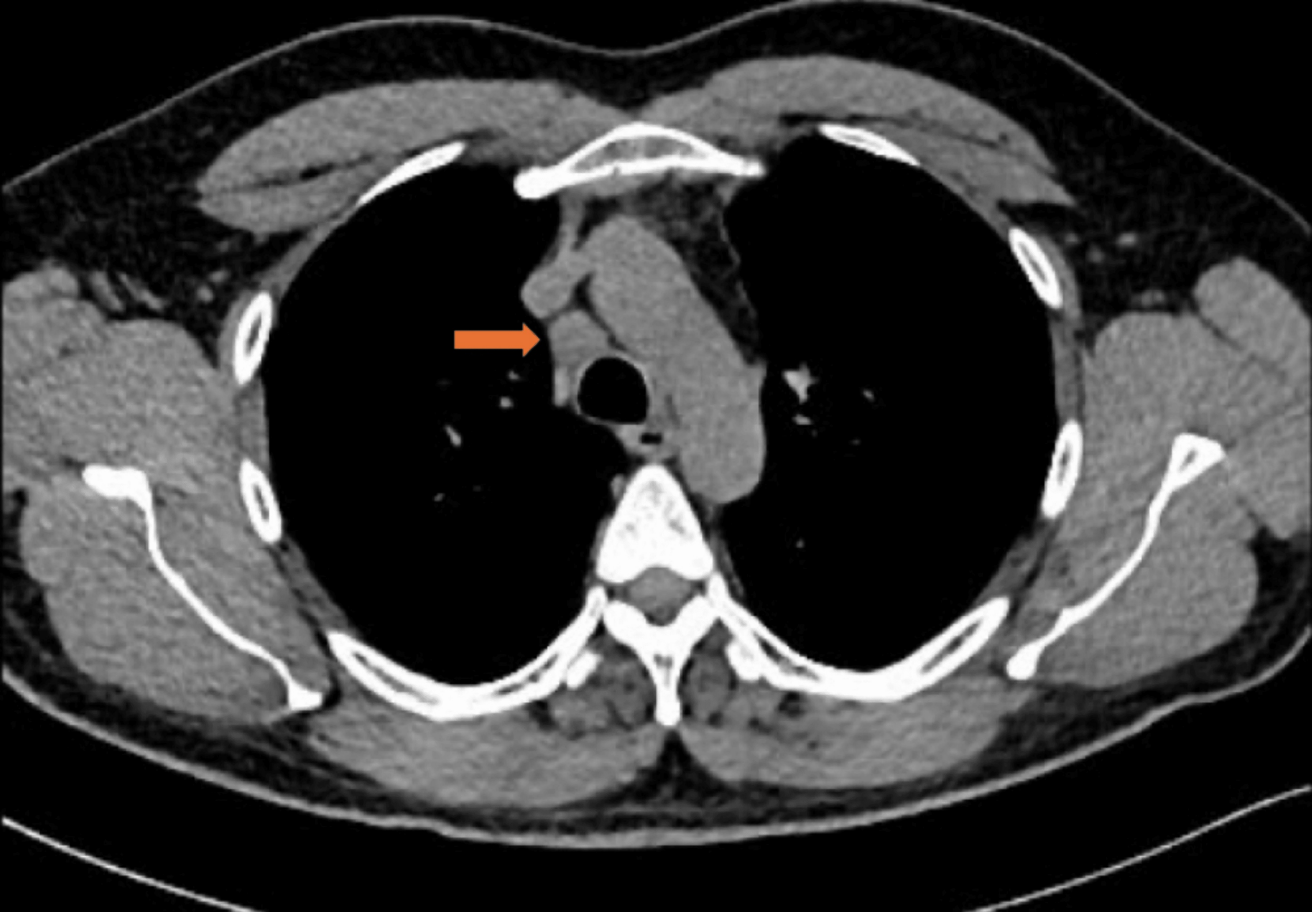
The axial image of the chest CT in the mediastinal window shows enlarged mediastinal (right lower paratracheal, station 4R) lymph node (arrow).
